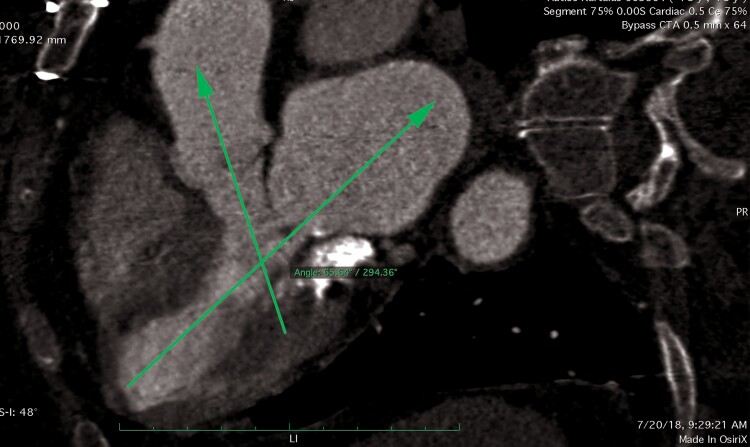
Aorto-mitral angle was measured 65/294° on multiplanar cardiac-gated computed tomography.
